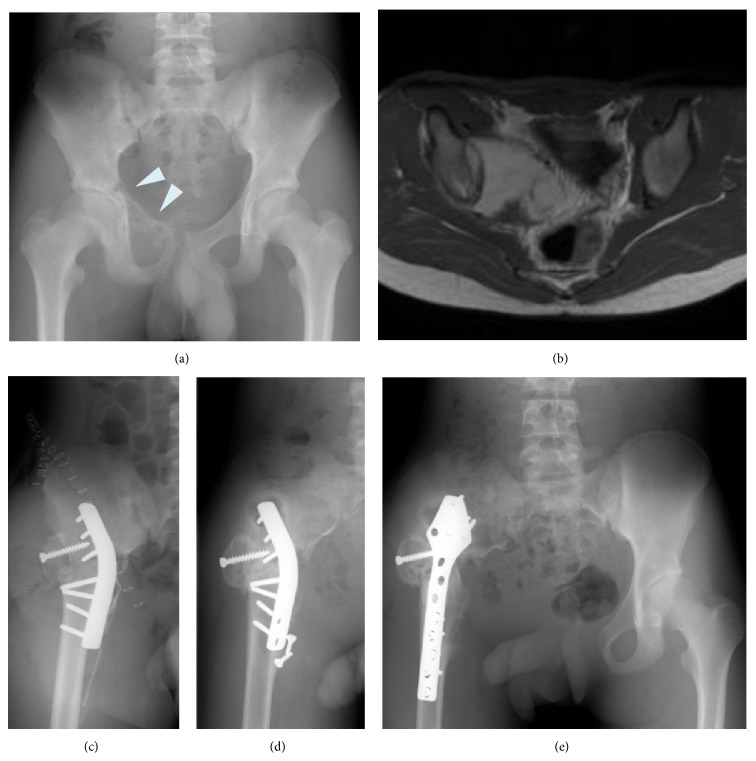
(a) Preoperative anteroposterior radiograph of the pelvis of a 14-year-old boy who had Ewing sarcoma of the acetabulum and pubis. (b) Axial T2-weighted MRI showing the tumor arising in the acetabulum. (c) Postoperative radiograph showing plate fixation of the proximal femur to the remaining ilium after PII-III resection. (d) Plain radiograph showing screw breakage 2 years after first iliofemoral arthrodesis. (e) Plain radiograph 11 years after refixation.
